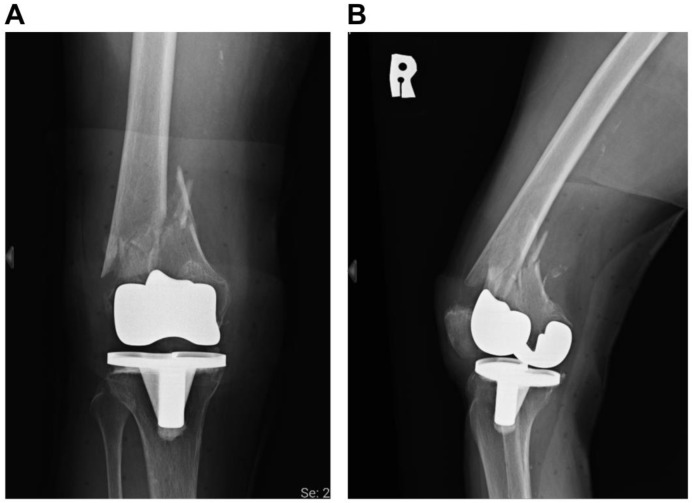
Preoperative radiographic images. (A) Anteroposterior (AP) view of knee. (B) Lateral view of knee. The patient was diagnosed with a periprosthetic distal femur fracture.
